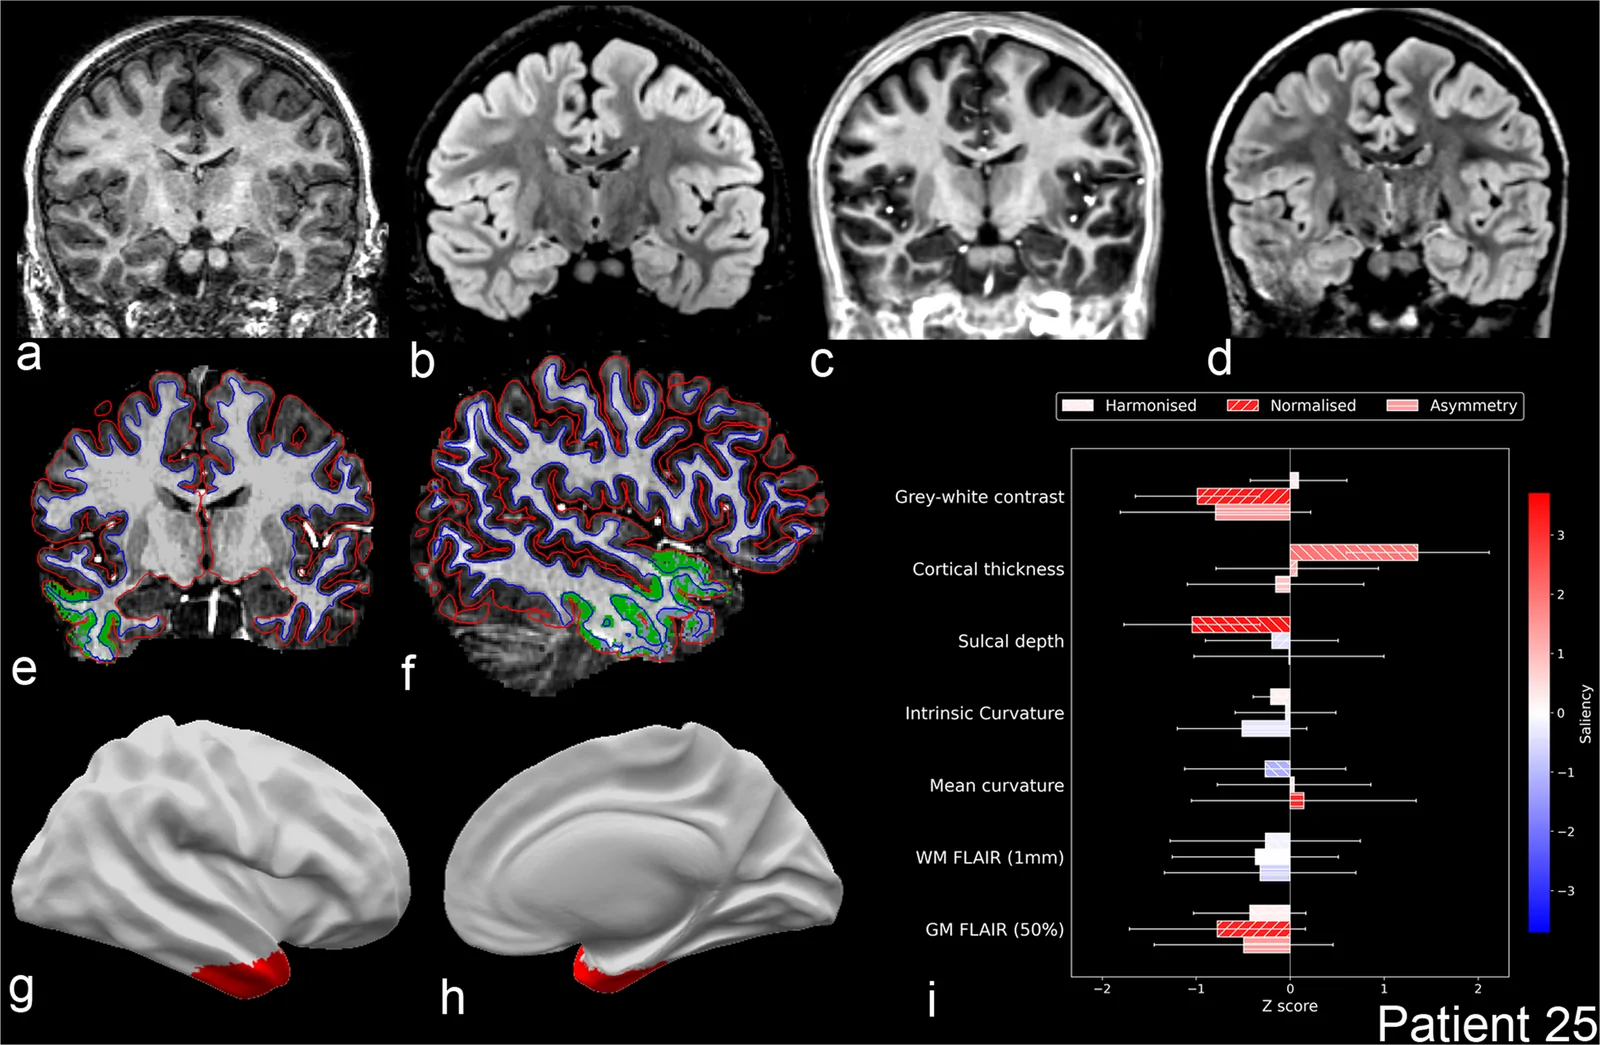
3 T and 7 T MRI, and 7 T segmentation and cortical surface-based reconstruction, and classifier results in a patient with a positive 3 T MRI (Patient 25). Coronal 3 T T1w (a) and FLAIR (b) MRI, and corresponding 7 T T1w (c) and FLAIR (d) MRI; coronal (e) and sagittal (f) 7 T T1w MRI after skull-stripping, with superimposed the lesion cluster detected by the 7 T classifier (green overlay) and *white* (blue line) and *pial* (red line) surfaces; 3D cortical surface models of the lateral (g) and mesial (h) right hemisphere with superimposed lesion cluster detected by the 7 T classifier (red overlay); 7 T classifier feature profile (i) of the lesion cluster
